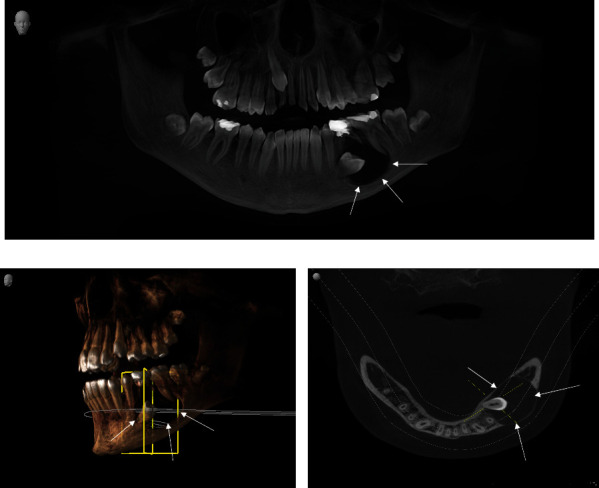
(a) OPG X-ray: unilocular radiolucency surrounding the crown of the impacted second premolar tooth #35, with its crown protruding into the cystis cavity. Border of the cystic lesion is in continuity with lamina dura of the tooth #75. (b) 3D CBCT: cystic lesion in relation to other anatomical structures of the mandible. (c) CBCT: coronal view of the cystic cavity.
